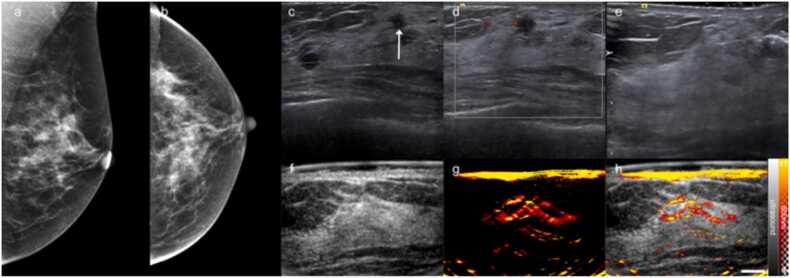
A false negative from the standard-of-care imaging that was successfully upgraded by OA-US. Mammography [MLO (a) and CC (b) views] do not indicate breast cancer (c) Standard-of-care ultrasound reveals a few scattered cysts and a small subcentimetre irregular hypoechoic mass in a retroareolar position. (d) Ultrasound imaging with Doppler demonstrates two small vessels. (e) Alternate view from ultrasound imaging without Doppler (f) The US image from the OA-US scan at the same position does not clearly reveal the irregular hypoechoic mass seen on US compared to the background breast parenchyma. (g) The OA image from the OA-US scan at 800 nm reveals a lattice of high signal similar to the irregular cap sign seen in malignancy, which would upgrade the lesion to BI-RADS 5. (h) Overlaid OA-US image. Scale bar 1 cm.
